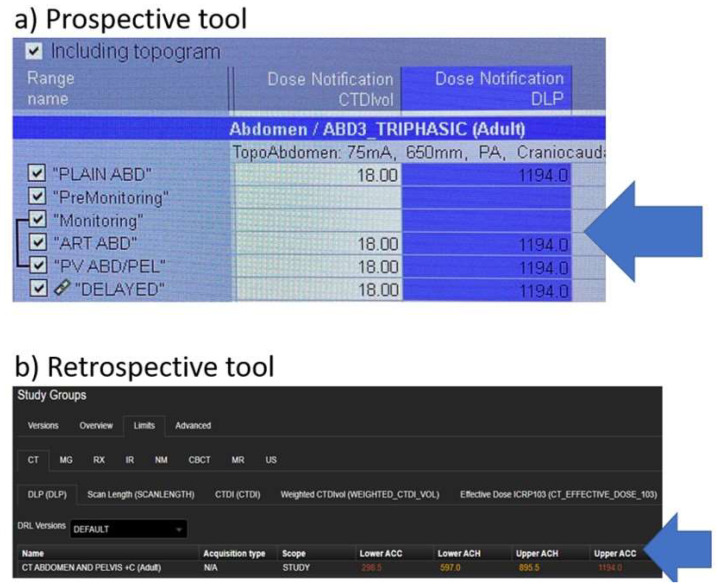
Demonstrates the key differences between the dose configuration setup for CT Abdomen/Pelvic + Contrast in a (a) prospective tool (CT system console) and (b) retrospective tool (DOSE TQM). a and b are screenshots taken straight from the CT system console and DOSE TQM, respectively.
